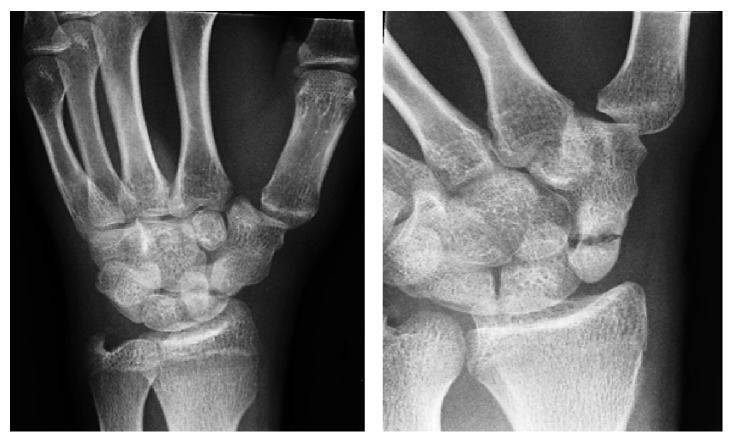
Radiographs show a displaced fracture through the waist of the scaphoid with Scaphotrapezial synostosis.
